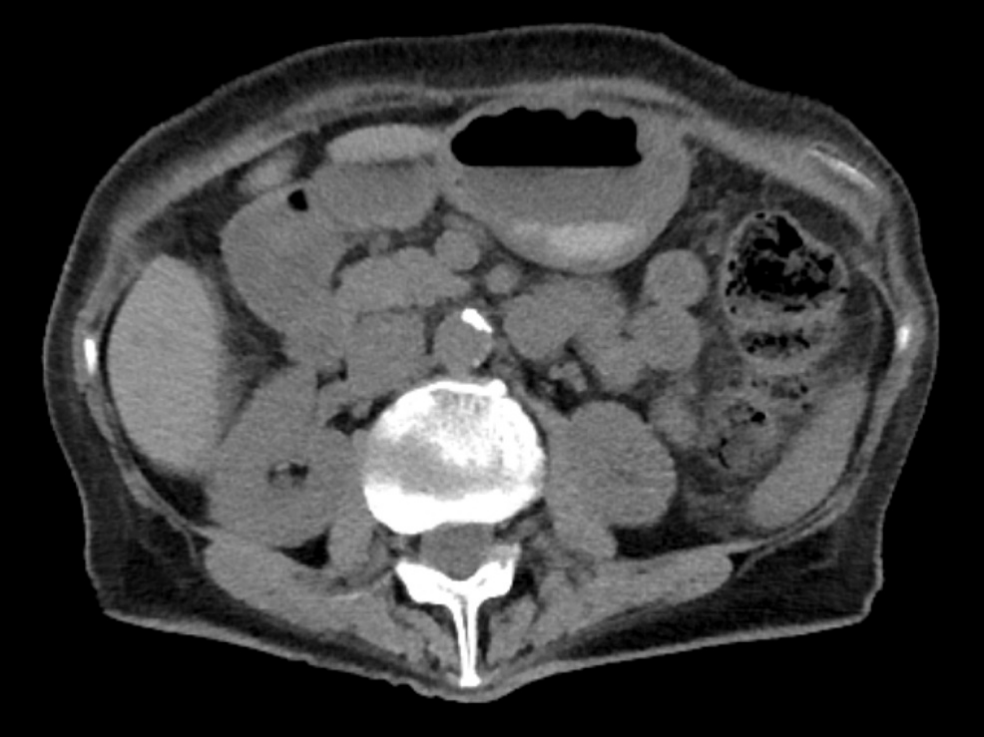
CT abdomen/pelvis demonstrates left renal atrophy with no hydronephrosis or nephrolithiasis.
